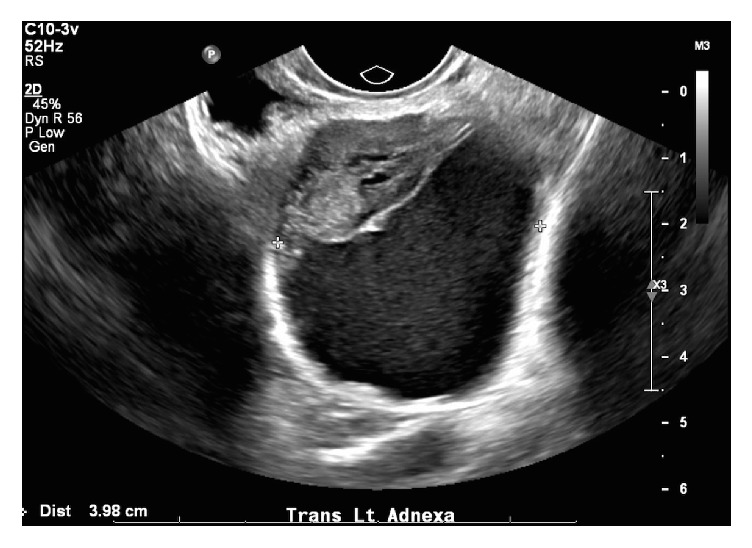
Ultrasound of the left adnexal mass in transview.
